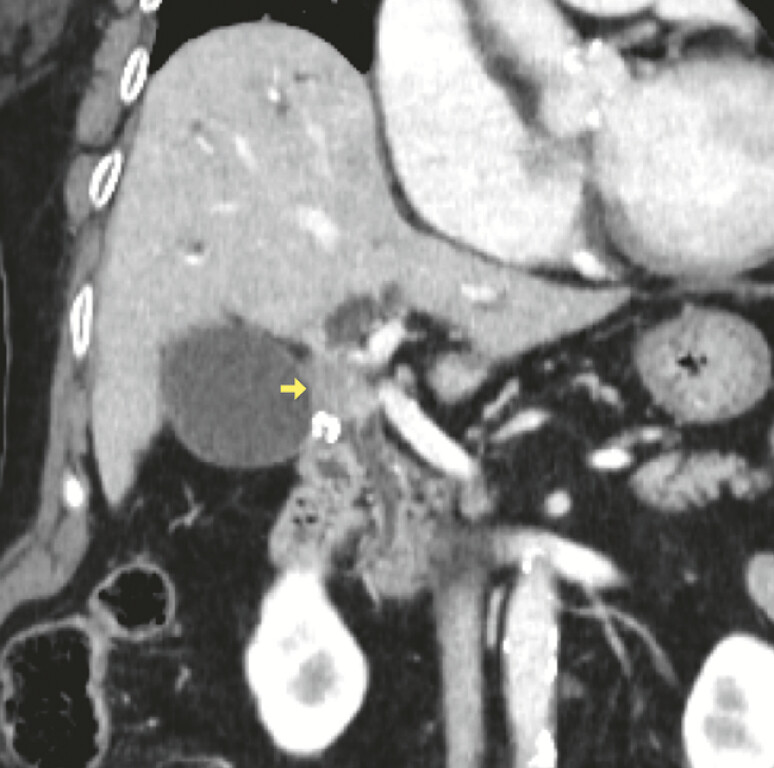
CT revealed a mass in the hilar bile duct region (yellow arrow).
